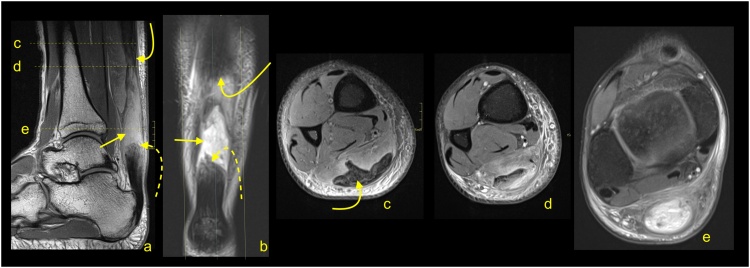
Total rupture of the midportion of the Achilles tendon (straight arrow), 3 weeks after trauma. MRI (a–e) showed that the proximal stump (curved arrow) and distal stump (dashed arrow) are frayed with significant diastasis. A and b- PD- weighted, c, d and e- PD- weighted with fat suppression.
